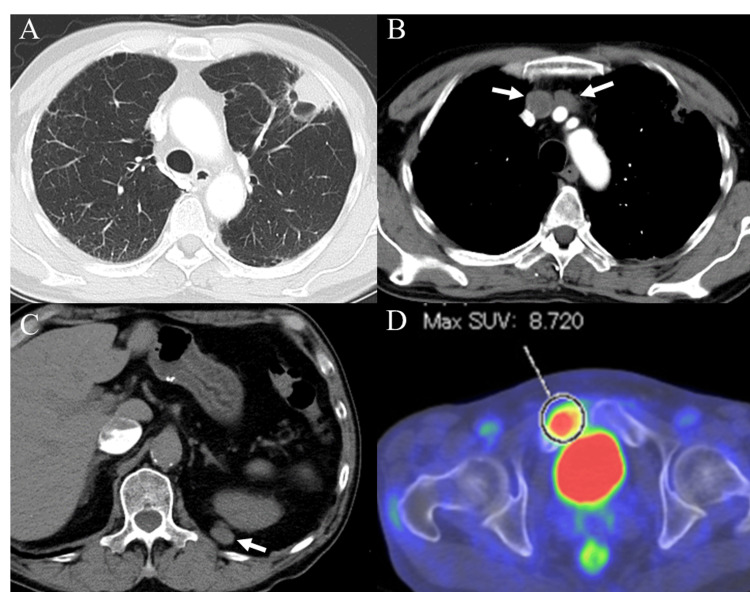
Initial imaging findings Contrast-enhanced whole body CT scan revealed a nodule in contact with the chest wall in the left upper lung lobe (A), multiple mediastinal lymphadenopathies (B, white arrows), and retroperitoneal metastases (C, white arrow). FDG PET/CT showed intense uptake of FDG in the pubic bone metastasis (D). FDG: F-18 fluorodeoxyglucose; PET/CT: positron emission tomography/computed tomography
